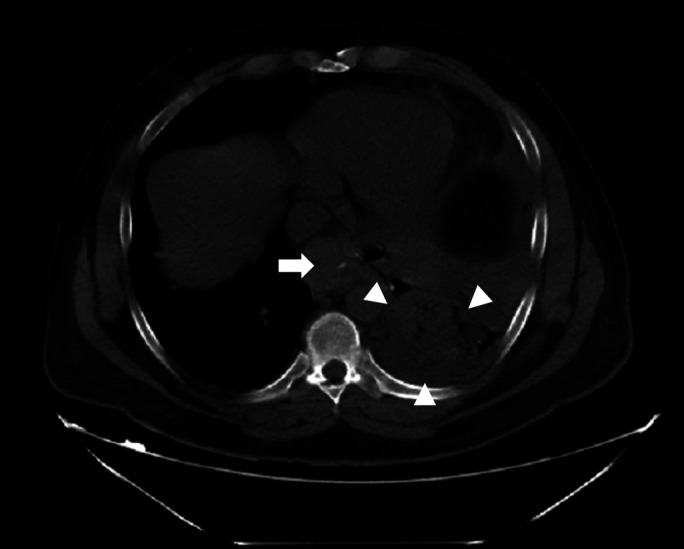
Noncontrast computed tomography scan demonstrating the gastric pouch (arrow) and the lung consolidation (arrowheads).
